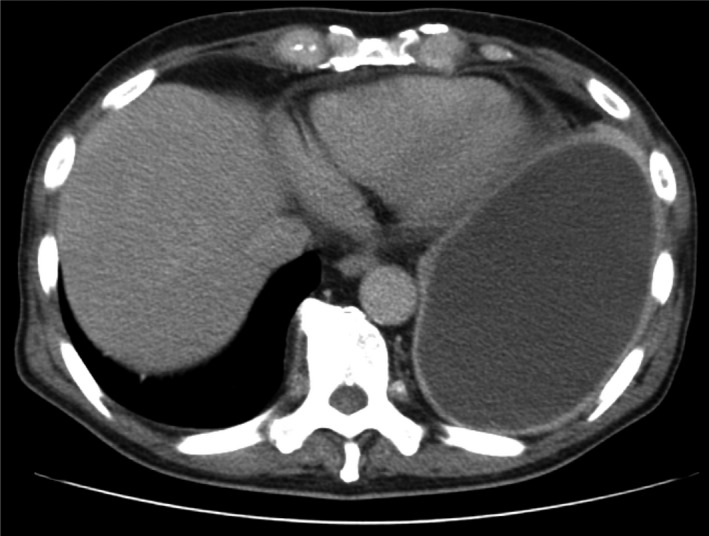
Enhanced chest computed tomography scan obtained at admission, showing extensive encapsulation of the left lung with thickened pleura
